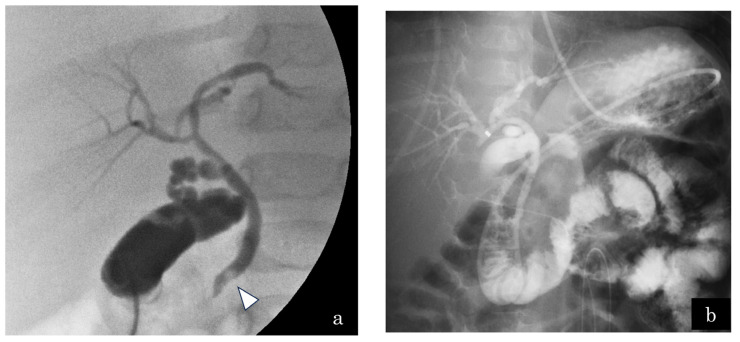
Case 7: A 5-month-old girl presented with gallbladder stones on ultrasonography. The patient developed septic shock due to cholangitis. (a) Emergency gallbladder drainage was performed, and a bile stone located in the distal common bile duct (white arrowhead) was observed. The pancreatic duct was not visualized by cholangiography. (b) A 4-Fr endoscopic nasobiliary drainage tube was inserted.
